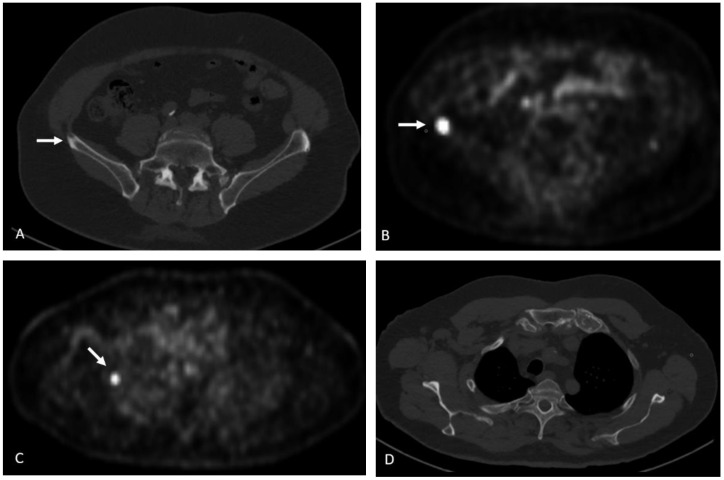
Seventy-four-year-old male with history of prostate cancer, status post-prostatectomy, now with rising PSA levels. NECT at the level of the sacrum (A) shows subtle sclerosis of the right iliac crest (arrow). 18F DCFPyL PET image (B) shows intense radiotracer uptake in the lesion (arrow). 18F DCFPyL PET image at the level of the scapula (C) shows radiotracer uptake within the right lateral 2nd rib (arrow) without a discernable abnormality on CT (D).
